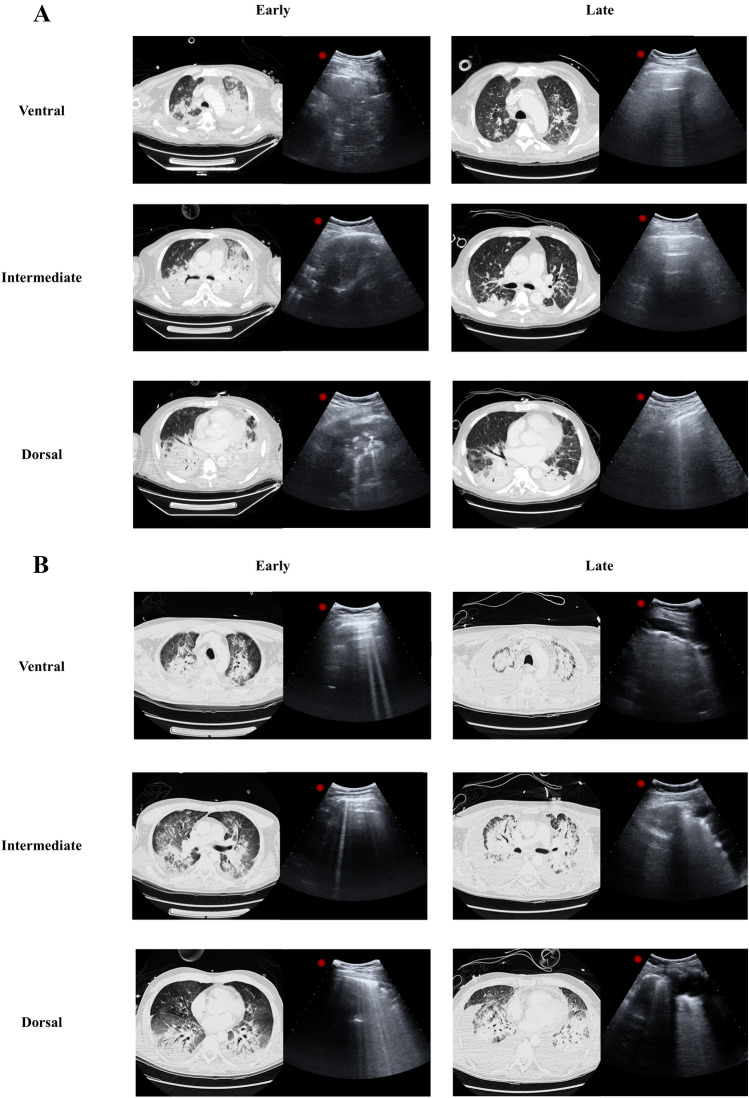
Representative lung CT and corresponding LUS images in ventral, intermediate and dorsal lung regions at early and late stages of ARDS, in two opposite cases of aeration improvement (panel A) or worsening (panel B)
